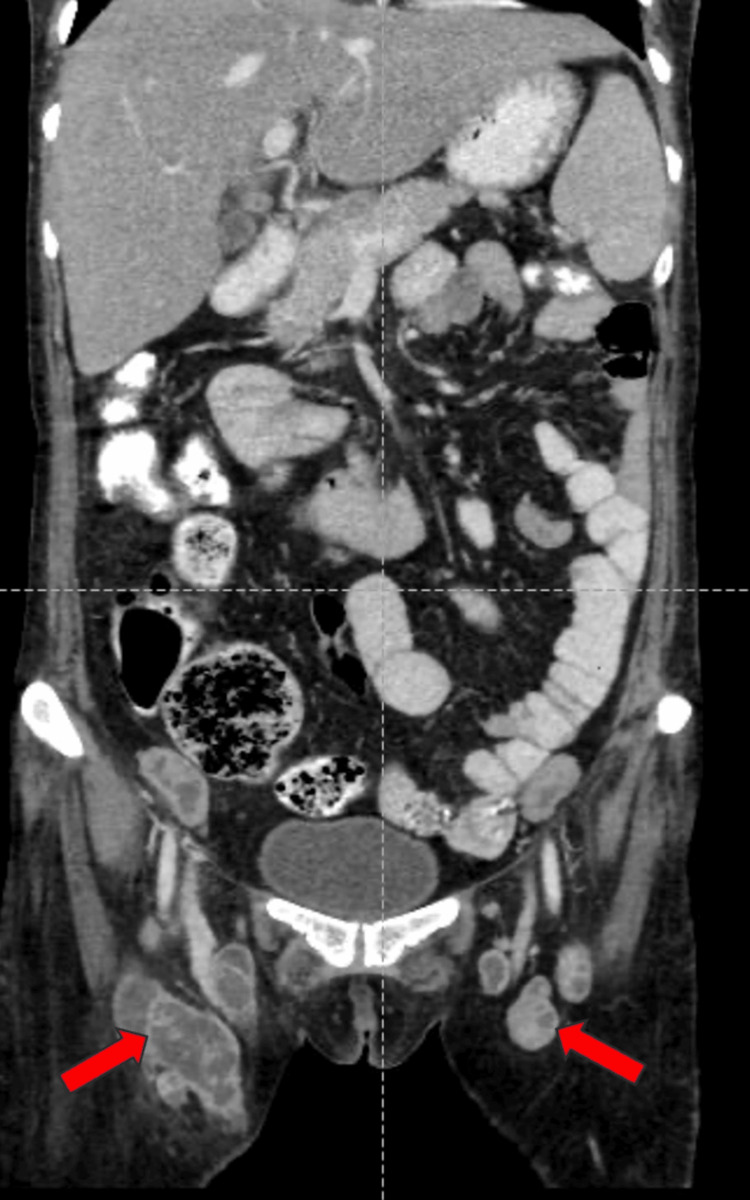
CECT-AP reformatted coronal section showing non-enhancing necrotic lymph nodes in the bilateral inguinal region shown by the red arrows. CECT-AP: contrast-enhanced computed tomography of the abdomen and pelvis
